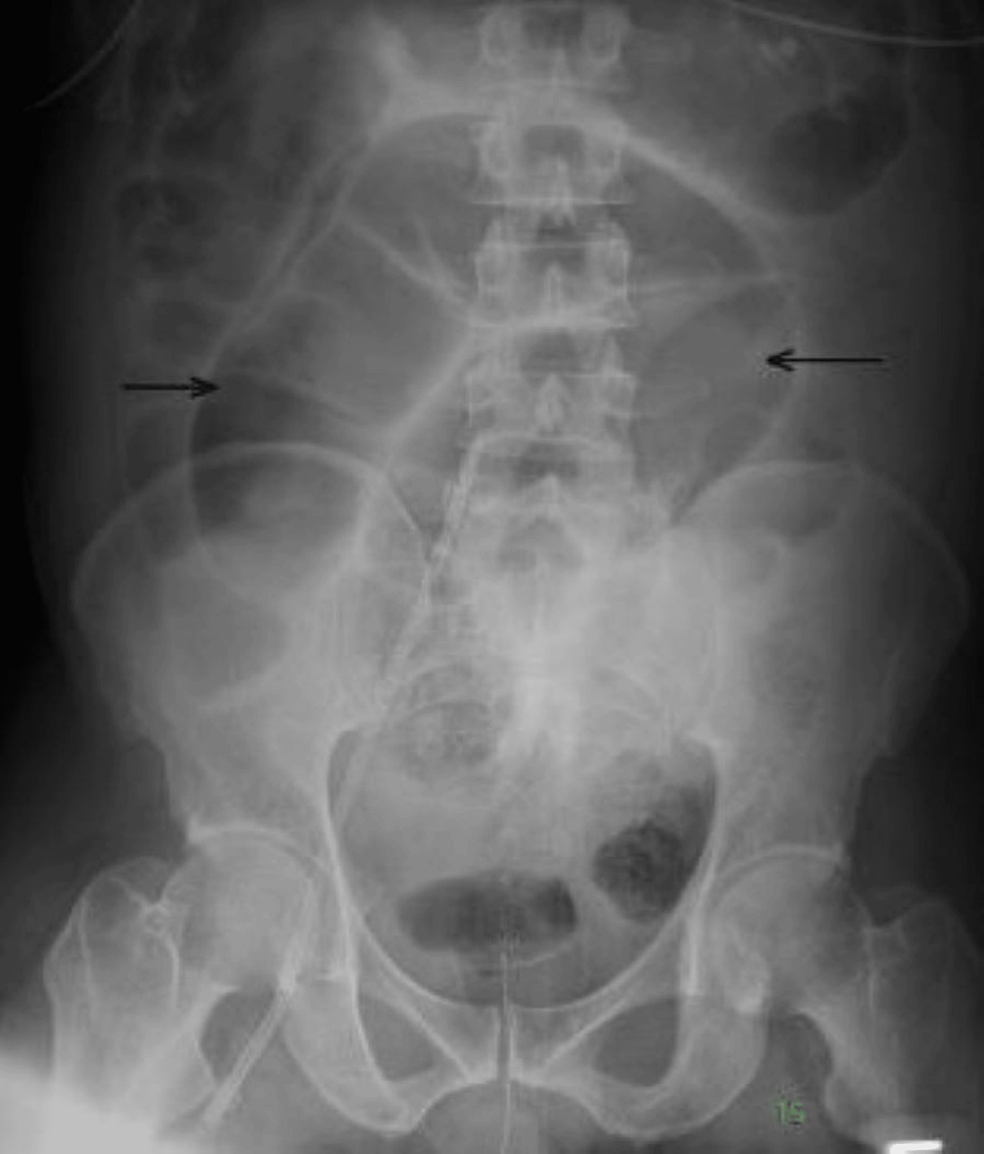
Abdominal X-ray showing the dilated colon
